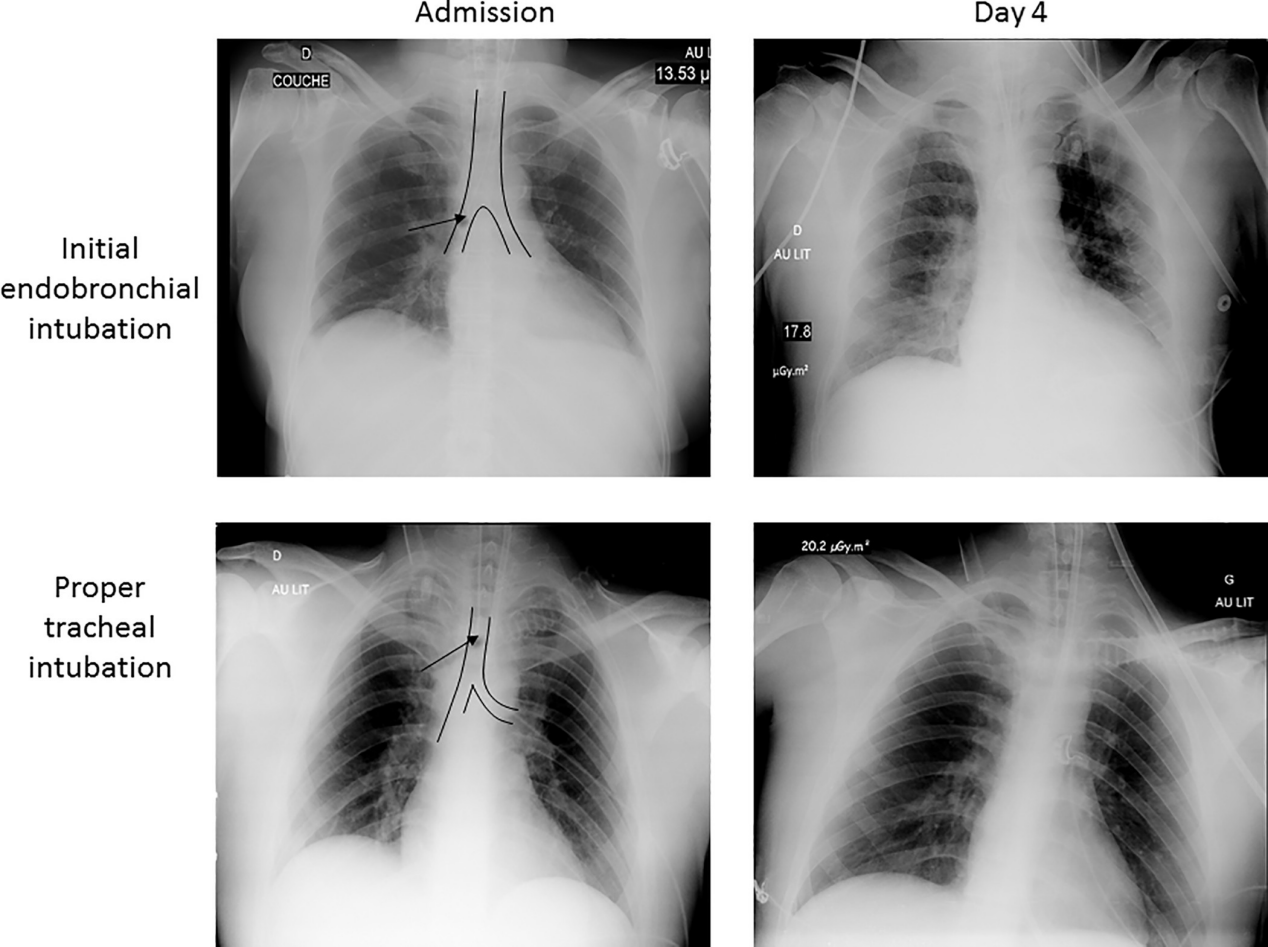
Chest X-ray obtained in a patient with an initial right endobronchial intubation and in another patient with initial proper tracheal intubation for comparison. The black arrow points to the tip of the endobronchial tube while the trachea and main right and left bronchi are underlined in black. On day 4, the patient with initial EBI developed basal lung infiltrates (predominant in the right base) compatible with VAP, whereas the patient with proper position of the tracheal tube on admission had an unremarkable chest X-ray.
